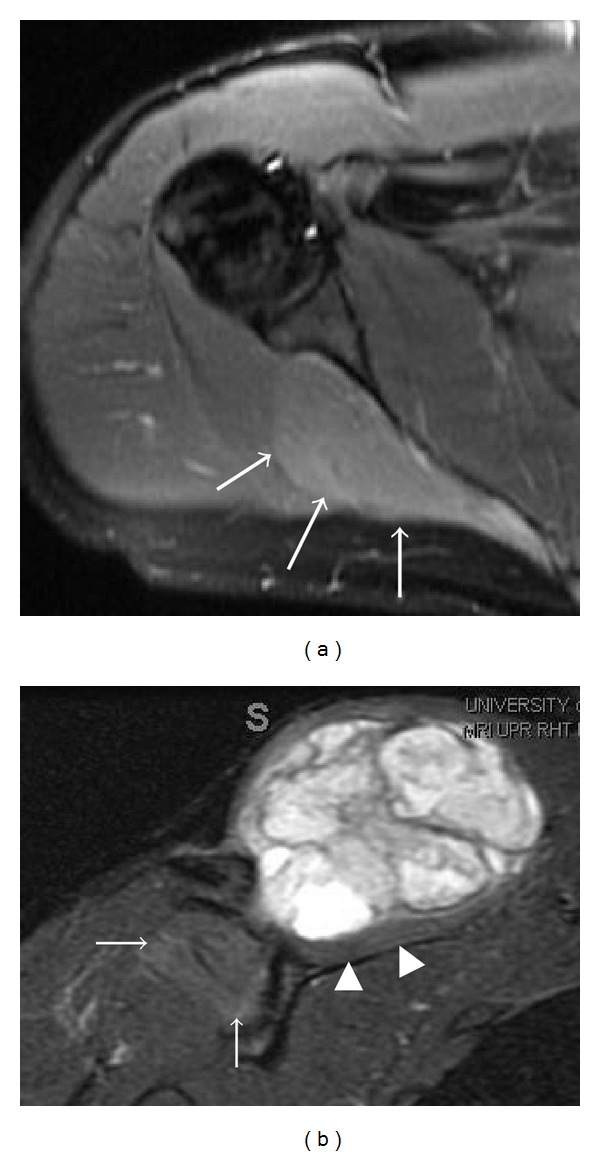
A high-grade sarcoma in a 28-year-old patient has MRI appearances indicating a right suprascapular nerve entrapment by the tumor at the spinoglenoid notch. Isolated increased signal intensity in the infraspinatus muscle (arrows) on axial (a) T2 fat-saturated image, with mild enhancement on coronal (b) postcontrast T1 fat-saturated image. The heterogeneous enhancing tumor produces mass effect on the supraspinatus muscle (arrowheads), which has normal signal intensity.
